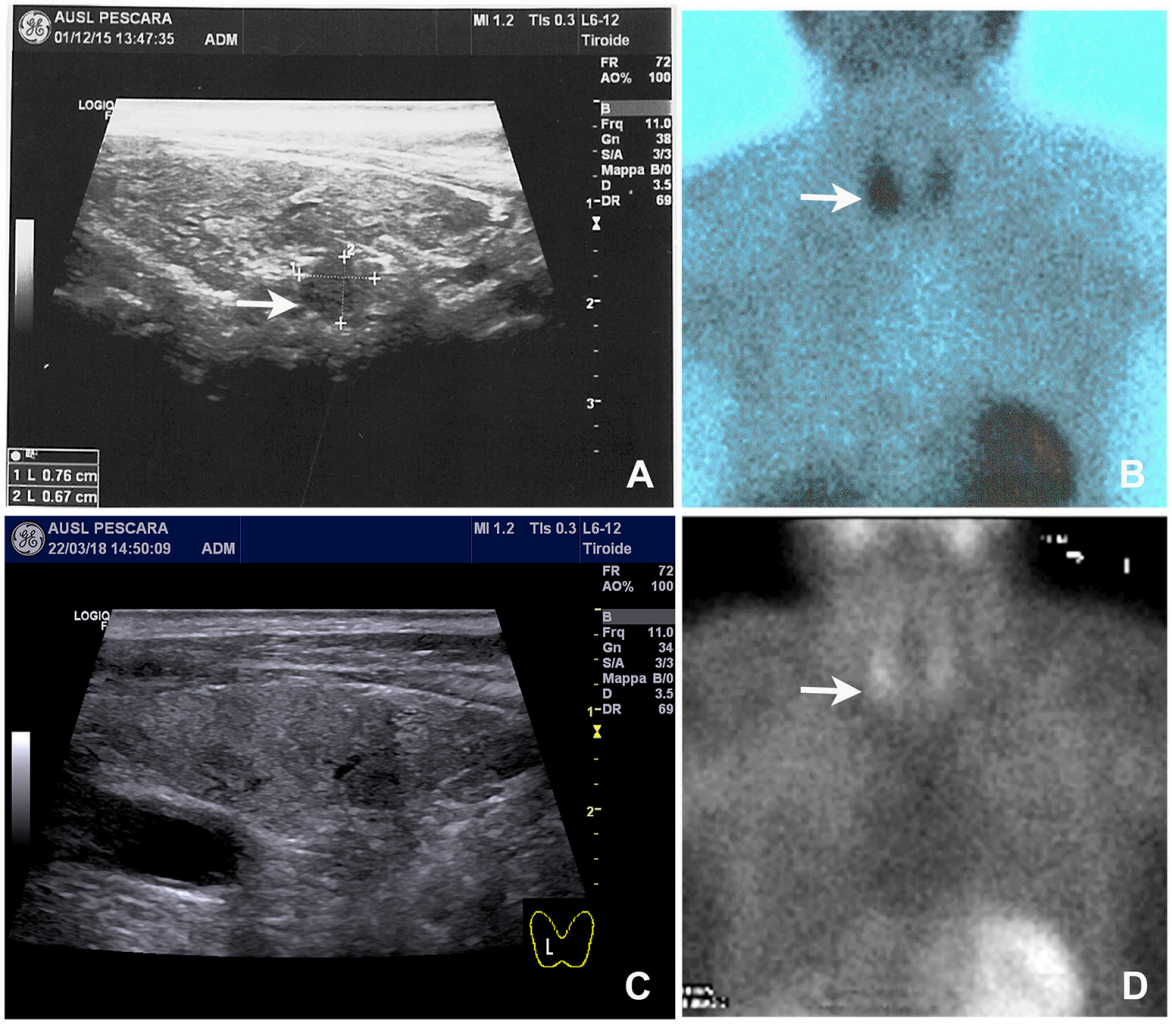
Neck ultrasound and ^99m^Tc-sestamibi scintigraphy performed on admission and 8 months after parathyroid apoplexy. (A) Baseline neck ultrasound showed a solid and hypoechoic 8 mm nodule located behind the right thyroid lobe (arrow). (B) Baseline ^99m^Tc-sestamibi scintigraphy showed an area of increased uptake (arrow). (C) Follow-up neck ultrasound performed 8 months later notice the near complete disappearance of the nodule. (D) Follow-up ^99m^Tc-sestamibi scintigraphy performed 8 months later showing a marked reduction of the uptake, although residual activity was still noticeable.
